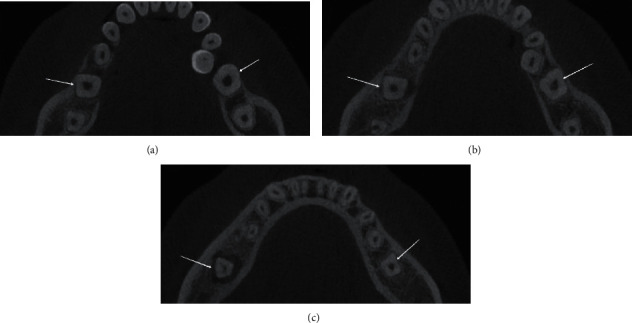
Axial section of CBCT scan showing the presence of a single canal in #36 and #46 at (a) cervical third, (b) middle third, and (c) apical third.
